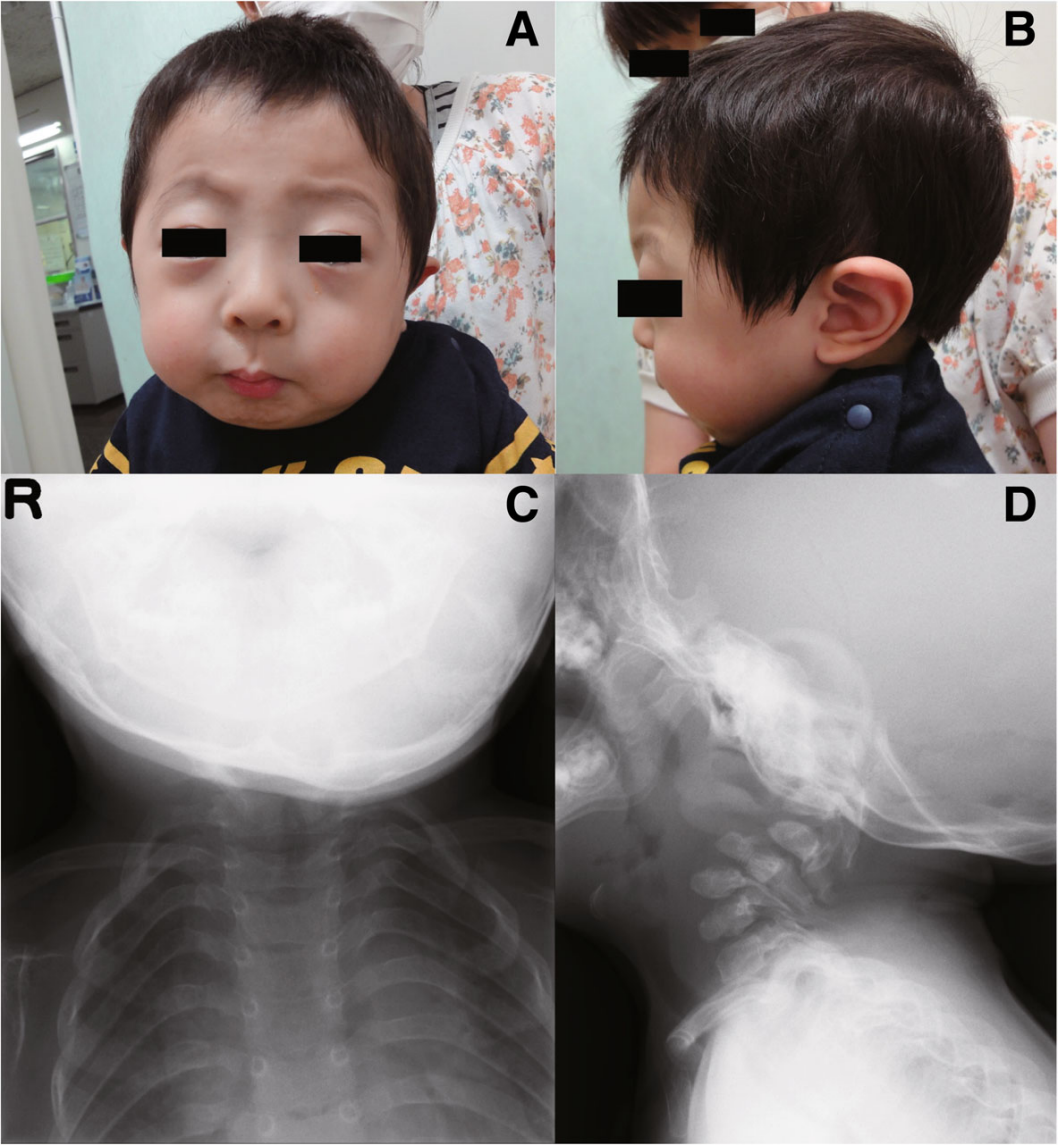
Facial photographs and X-ray films of the patient. a, b The facial photographs show the typical facial features associated with Schwartz–Jampel syndrome. Fixed facial expression, micrognathia, and low-set ears. c, d X-ray films show cervical kyphoscoliosis
